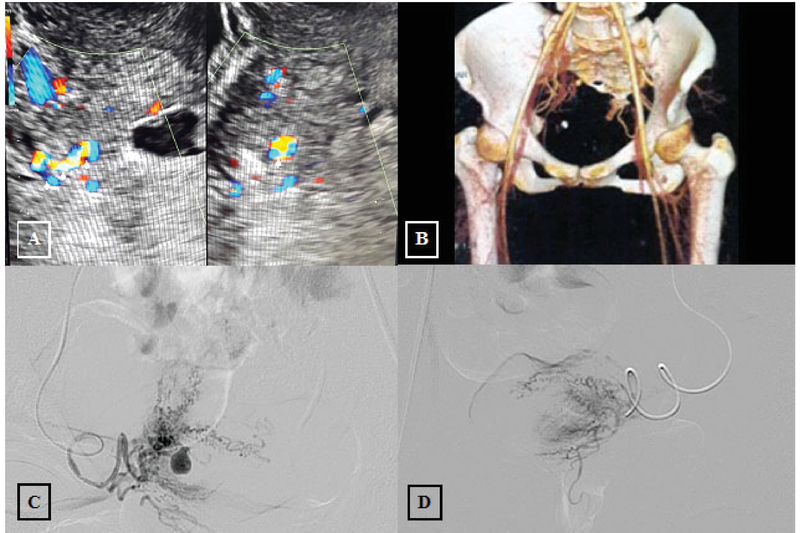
(A) New vascularization on the cesarean scar with vessel enlargement; (B) Lobulated oval lesion on CT Angiography; (C) Aneurism at the right ascending uterine artery; and (D) TAE procedure found that there were no aneurism and hypervascularization.
